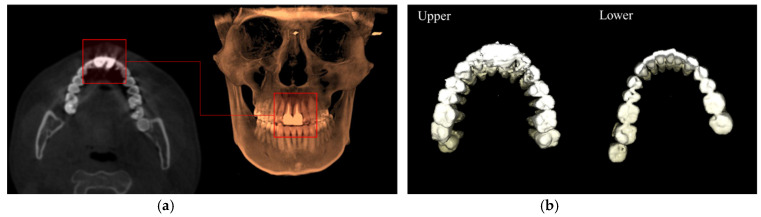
Example for solved CBCT cases with a metal artefact: (a) Metal artefact; (b) 3D upper/lower segmentation.
